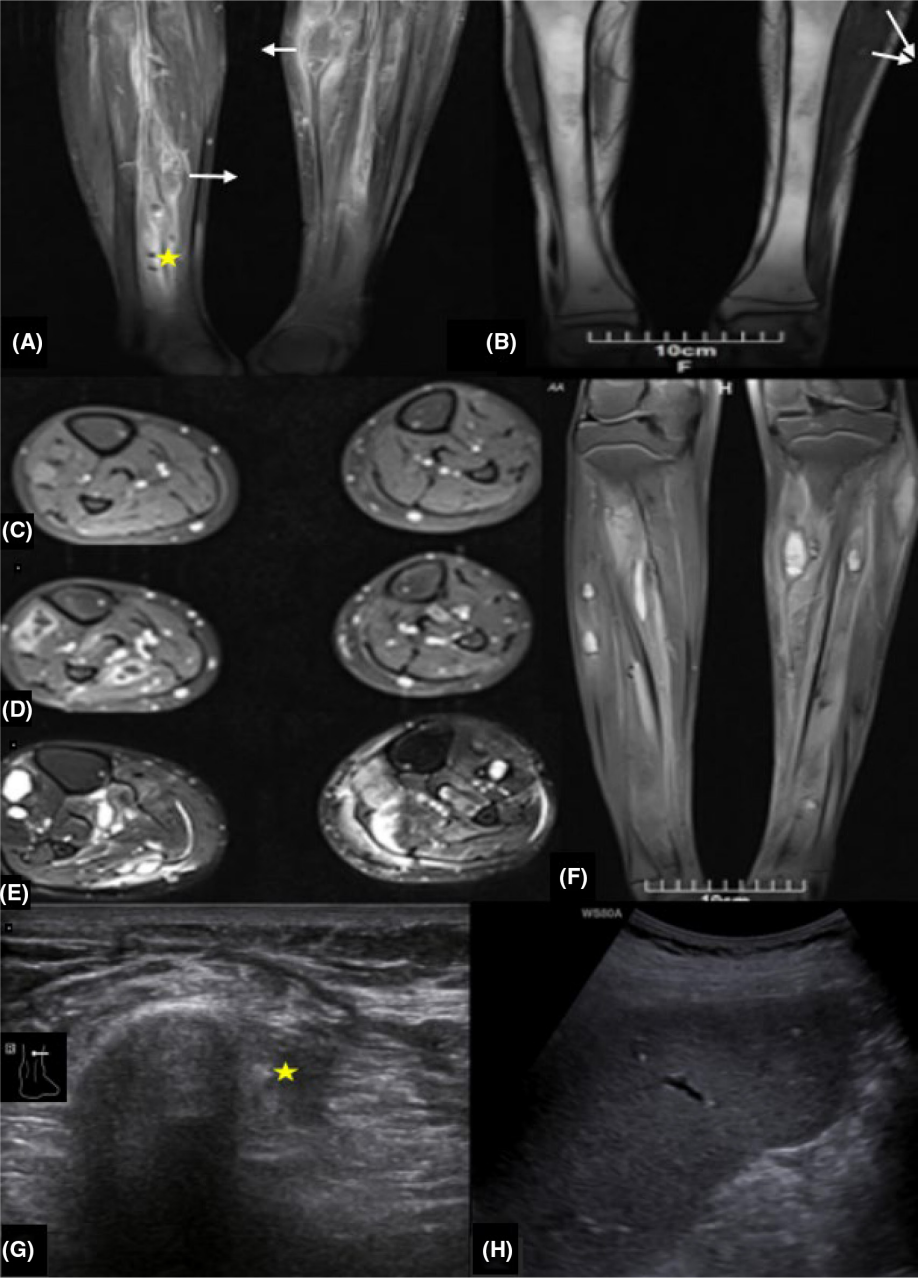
Post‐treatment MRI and ultrasound. After the antifungal treatment, some of the soft tissue abscesses coalesced with each other, some of the lesions disappeared, and some of them calcified. Coronal T1 fat‐sat sequence with contrast shows ring‐enhancing oval‐shaped lesions in the medial muscular compartment of the legs (white arrows). Surrounding edematous tissues also show enhancement. Some foci were signal void on all pulsed sequences and did not show any enhancement (yellow star) (A). Coronal T1 weighted sequence without contrast shows complete resolution of bone marrow infiltration. Two small, oval‐shaped, iso‐ to hypersignal lesions are seen at the lateral muscular compartment of the left leg (white arrows) (B). Axial T1 fat sat without contrast (C), axial T1 fat sat with contrast (D), and axial T2 fat sat images (E) show multiple iso to hypersignal T1 weighted, hypersignal T2‐weighted, oval‐shaped lesions in different muscles of legs, accompanied with ring enhancement. Coronal PD fat sat sequence (F) shows similar hypersignal lesions. Signal void foci on MRI (yellow star in A) were echogenic with faint acoustic shadow in the ultrasound exam (G), indicating calcification (a sign of response to treatment). Hypoechoic splenic lesions (Figure 1A) were calcified after the treatment (H).
